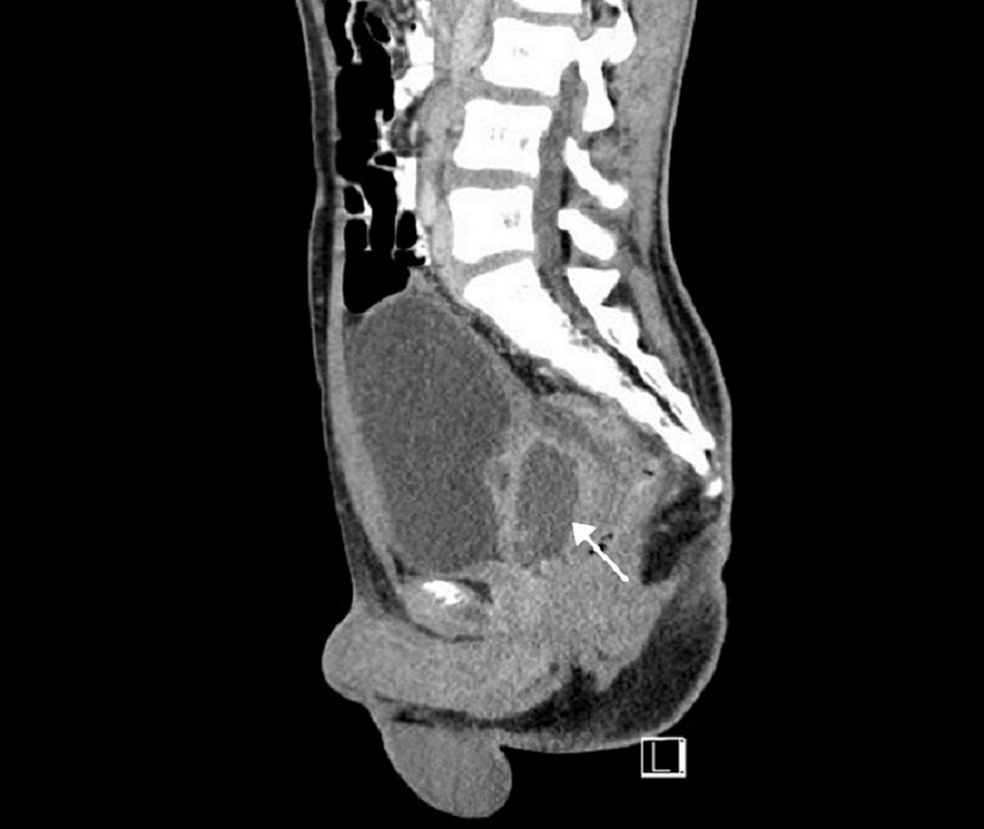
Computed tomography (sagittal view) revealing cystic lesion compressing on the posterior bladder wall
